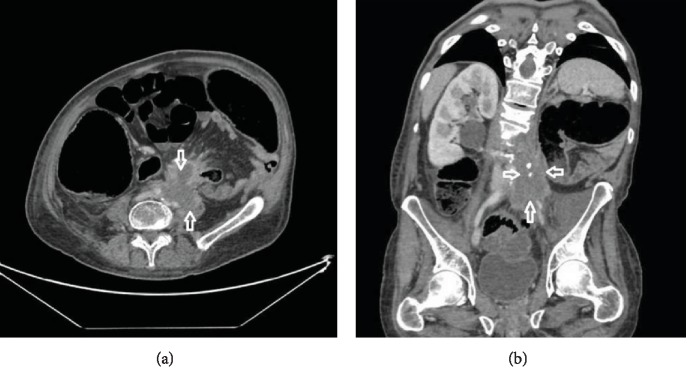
Abdominal CT scan showing a left lateroaortic mass (a) spanning over 12 cm and infiltrating the left psoas and iliac muscles, as well as the left iliac vessels (b).
